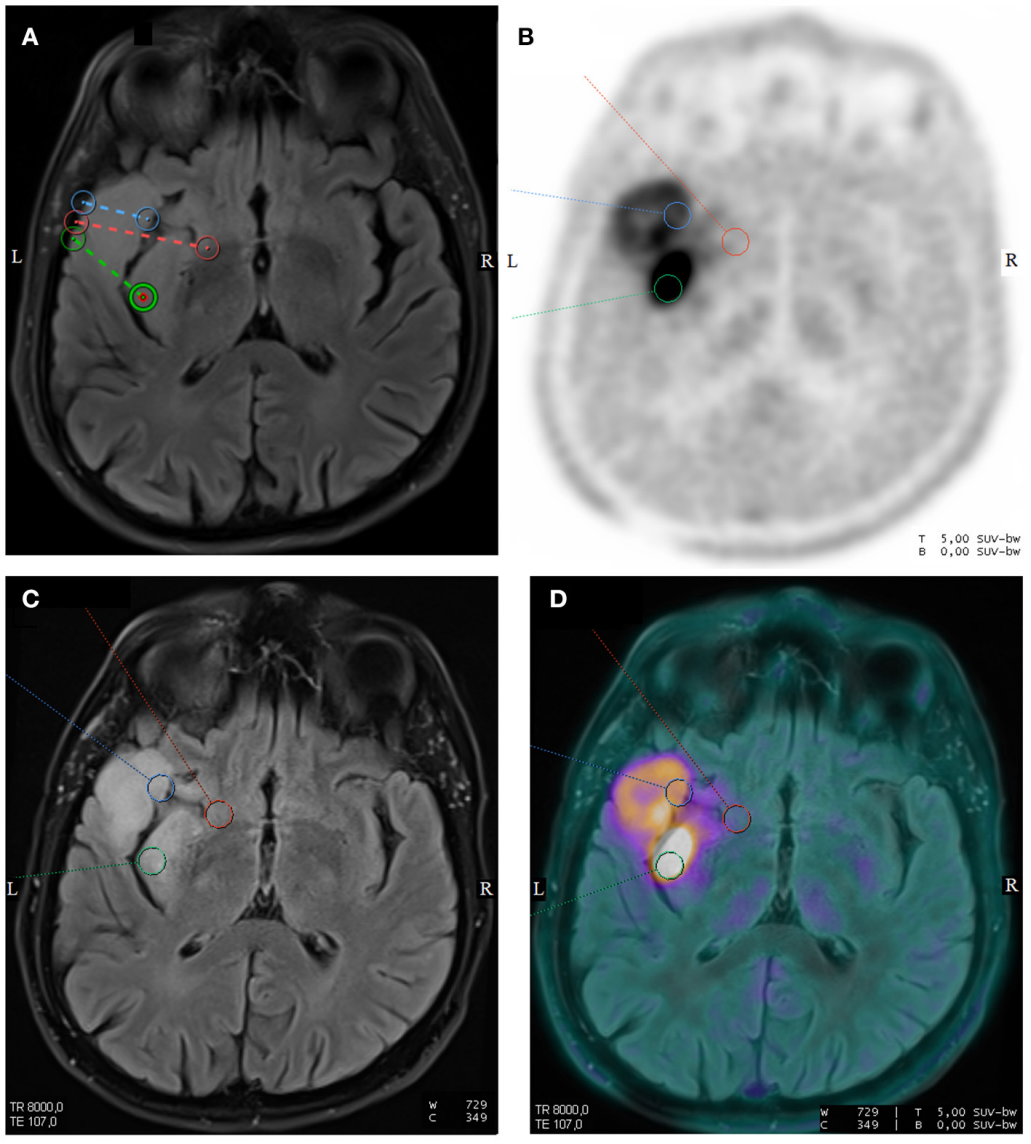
Axial T2-weighted fluid-attenuated inversion recovery (FLAIR) sequence from surgical navigation software with the locations of three neuronavigation-guided biopsies in a 59-year-old man with a left temporal diffuse IDH1-wild-type glioblastoma (A). Corresponding axial images of FDOPA PET (B), T2-weighted FLAIR sequence (C), and fused FDOPA PET/T2-weighted FLAIR sequence (D) are displayed. The pathology grading of the neuronavigation-guided biopsies revealed WHO grade II for the red VOI and WHO grade III for the green and blue VOIs.
